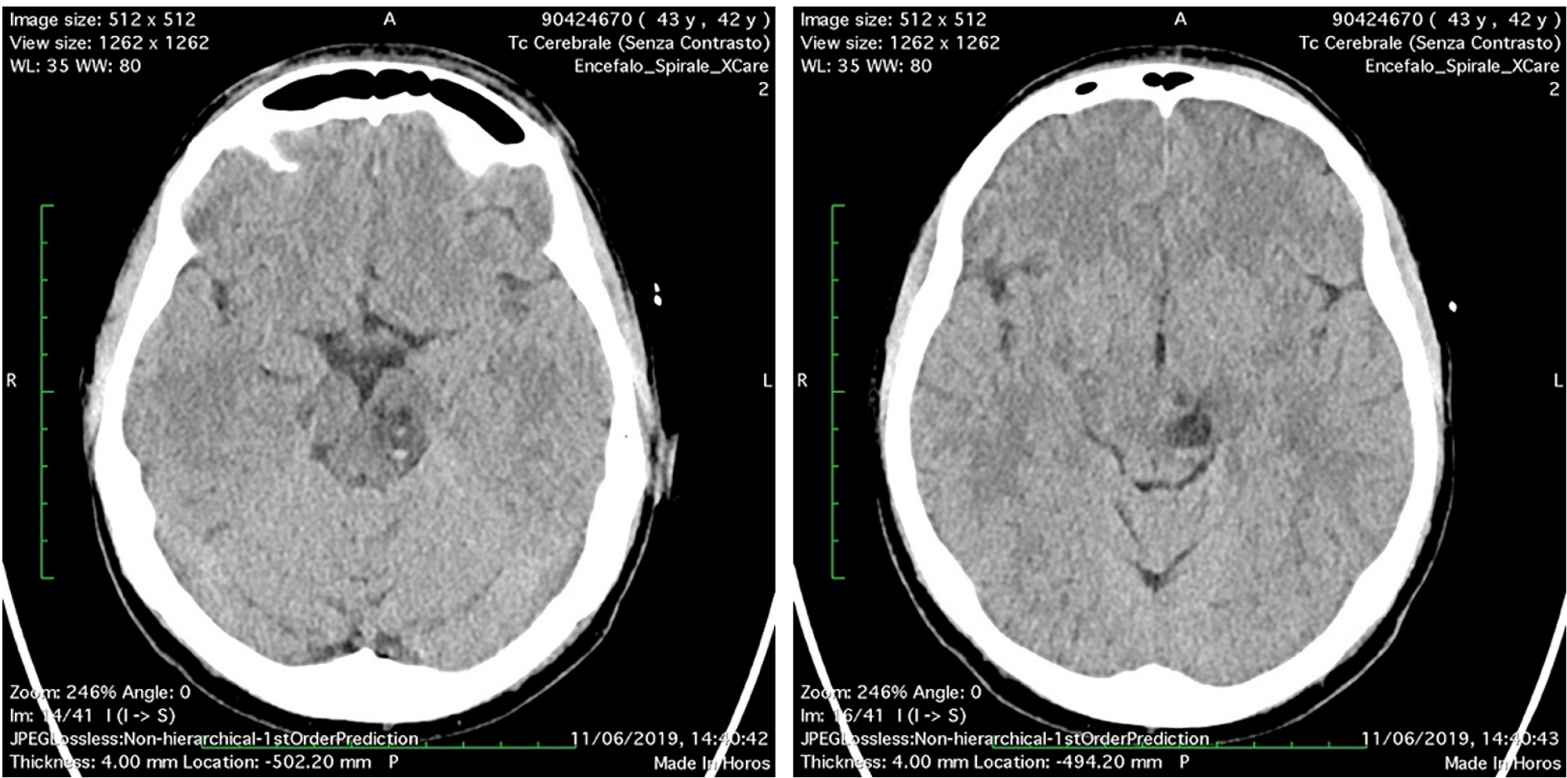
(A-B) Head CT scan “two days after”: no significant changes of the bleeding PVSs in the left midbrain. PVSs, perivascular spaces
